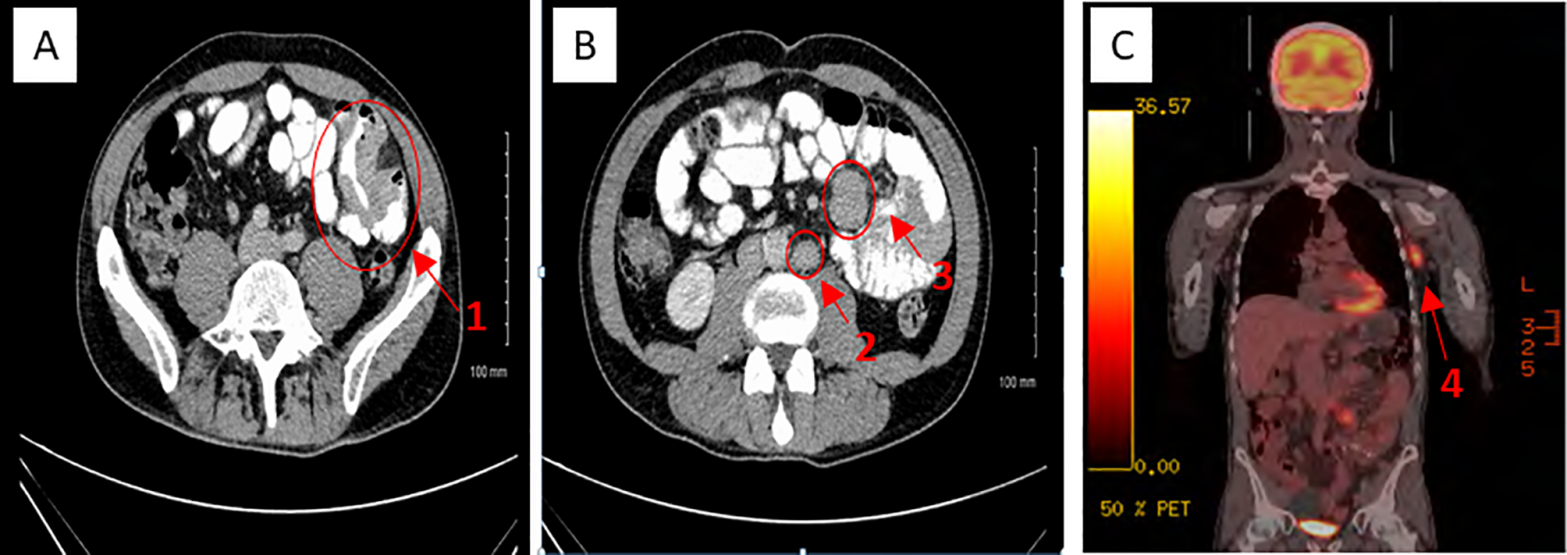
CT (A, B) and PET-CT (C) appearances of metastatic jejunal melanoma. 1 = Metatstatic jejunal small bowel obstruction. 2 = Retroperitoneal lymphadenopathy. 3 = Multifocal small bowel mesenteric melamona. 4 = Metastatic left axillary lymphadenopathy.
